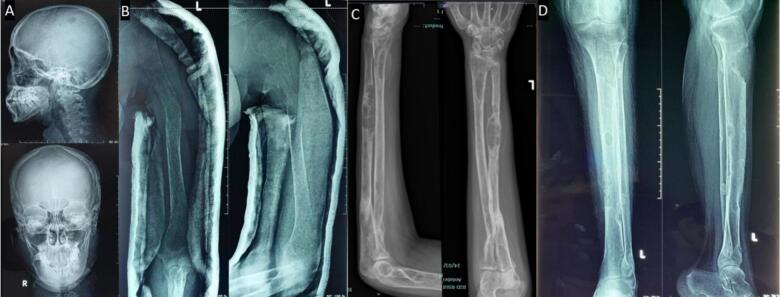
X-ray radiograph of our patient with brown tumor, showing multiple osteolytic lesions with cortical thinning in the skull (A), humerus (B), radius, ulna (C), and tibia (D) characteristic of hyperparathyroidism.
